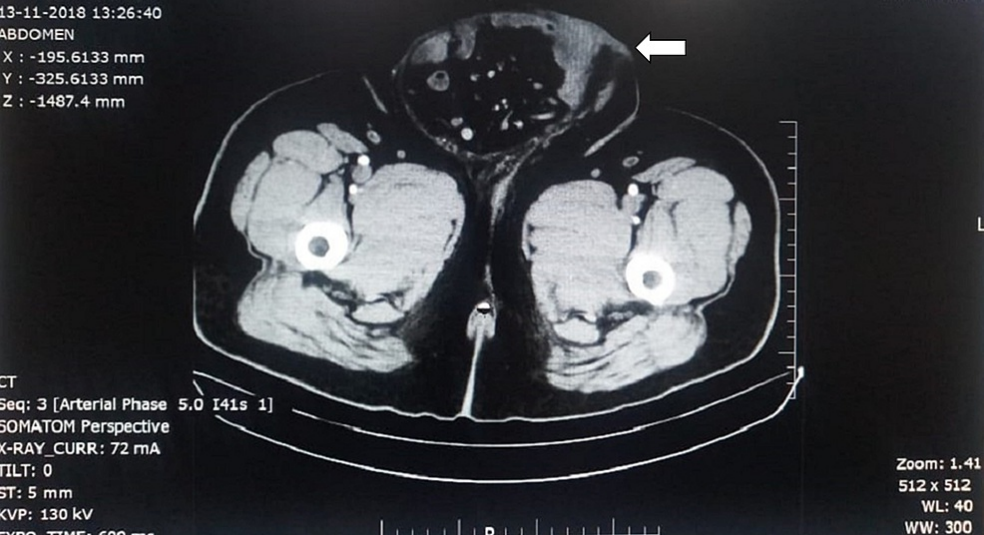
CECT abdomen showing large inguinal scrotal hernia with enterocele at mid-thigh level CECT- Contract-enhanced computed tomography
